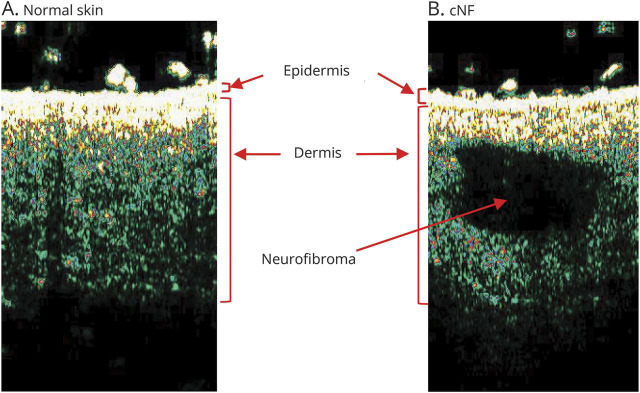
High-frequency ultrasound of skin Comparison of normal skin (A) to skin with cutaneous neurofibromas (cNF) (B) using a 20-MHz transducer on the DermaScan C ultrasound. The neurofibroma is hypoechoic.
